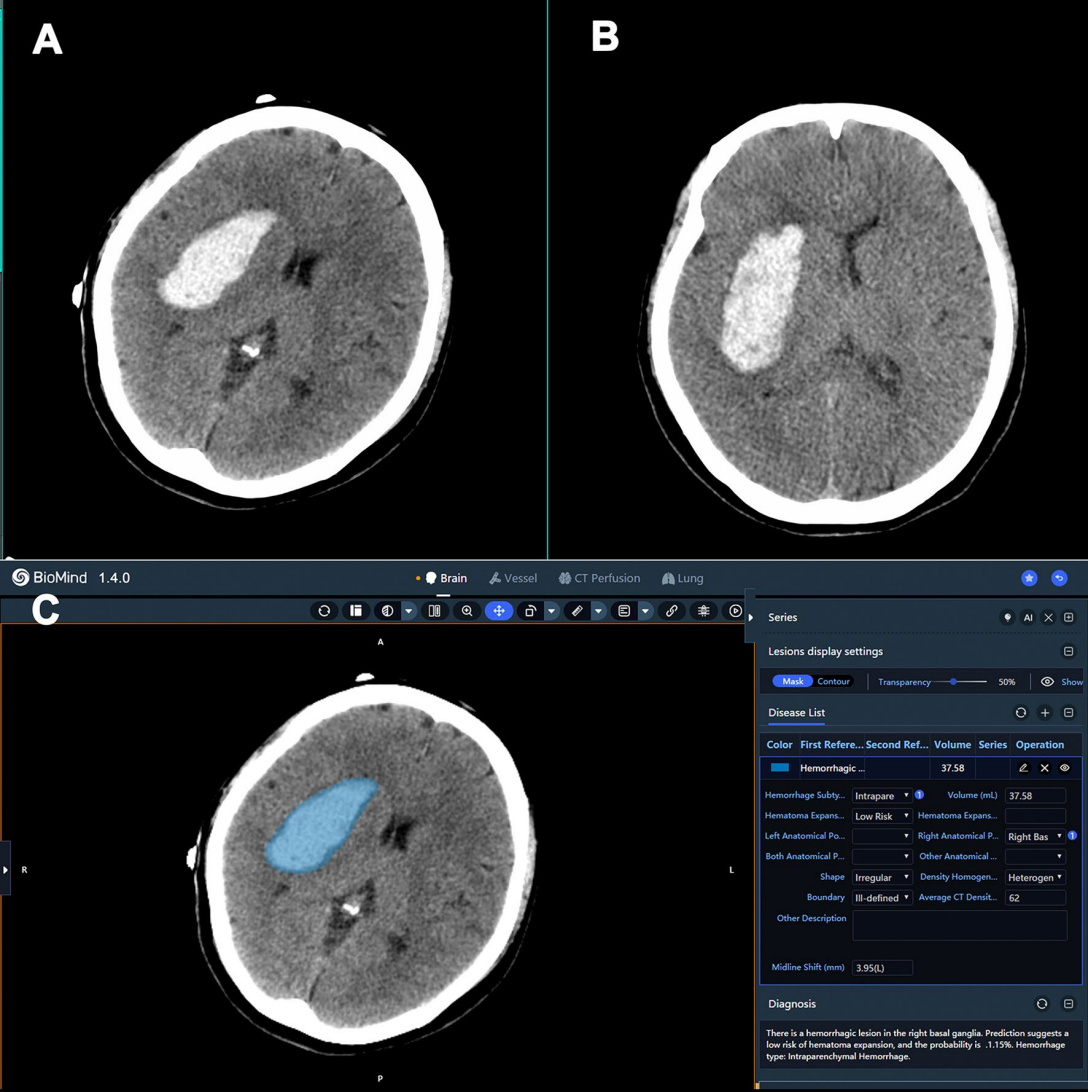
A Initial CT scan image of a 51-year-old female HICH patient. The patient was diagnosed with hematoma expansion by the doctor according to CT signs. B Follow-up CT scan within 6 h after the initial scan, the hematoma volume increased by 12.9 ml, and was confirmed as hematoma expansion. C AI software automatically analyzed the initial CT scan image of the patient after onset. The blue colored mask refers to the delineation of hematoma and the prediction of hematoma expansion by AI software was shown in the right side
